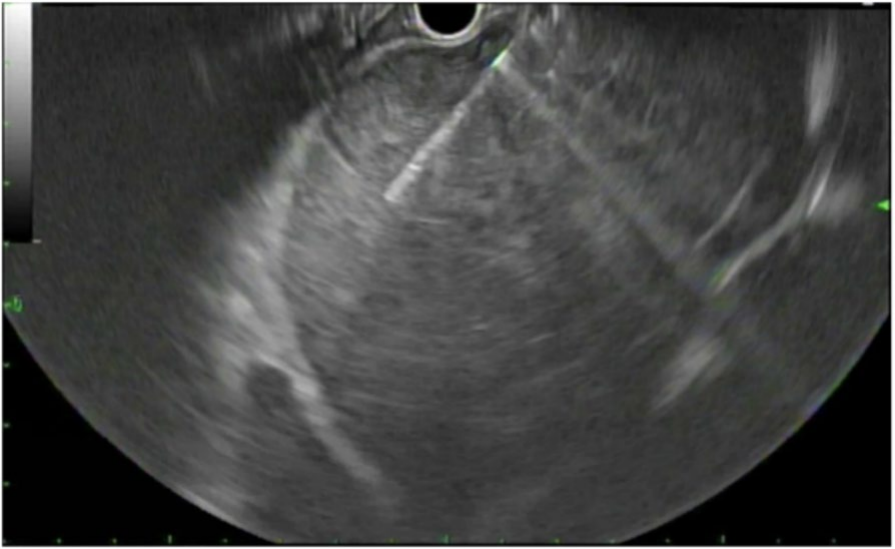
Upper endoscopic ultrasound-guided fine needle aspiration of the cardiac tumor
